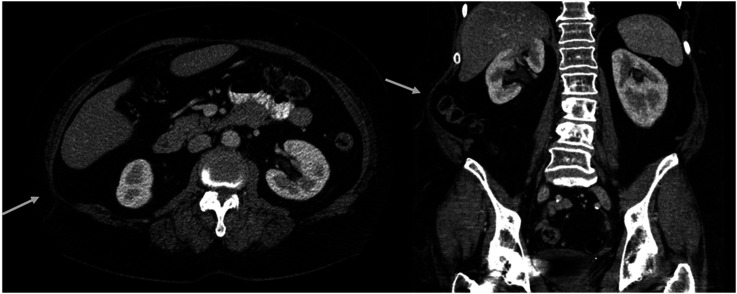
Example of bulge score 2 in axial and coronal reformatted CT images. High-grade focal atrophy in right upper posterior abdominal wall, graded as bulge score 2 by both observers.
